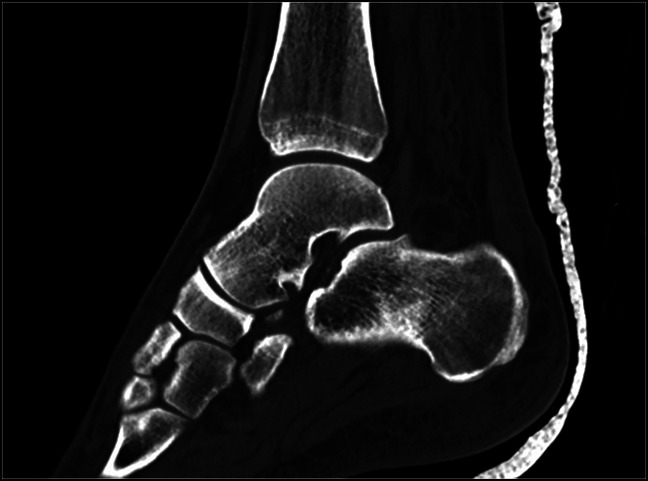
Immediate postreduction sagittal CT image showing realignment of the ankle, subtalar, and talonavicular joints.
